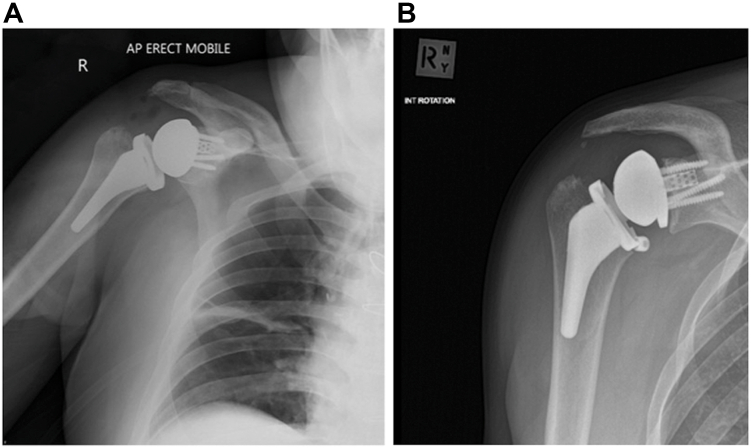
(A) Immediate postoperative radiographs, anteroposterior view, with clear stepoff. (B) 6-week postoperative radiograph with broken screw head and dissociation of the glenosphere. *AP*, anteroposterior.
